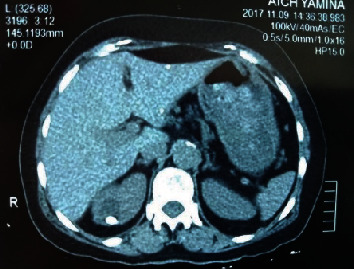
CT demonstrates a 10 mm stone-bearing diverticulum with 750 UH of density situated in the pole with the holmium laser.
